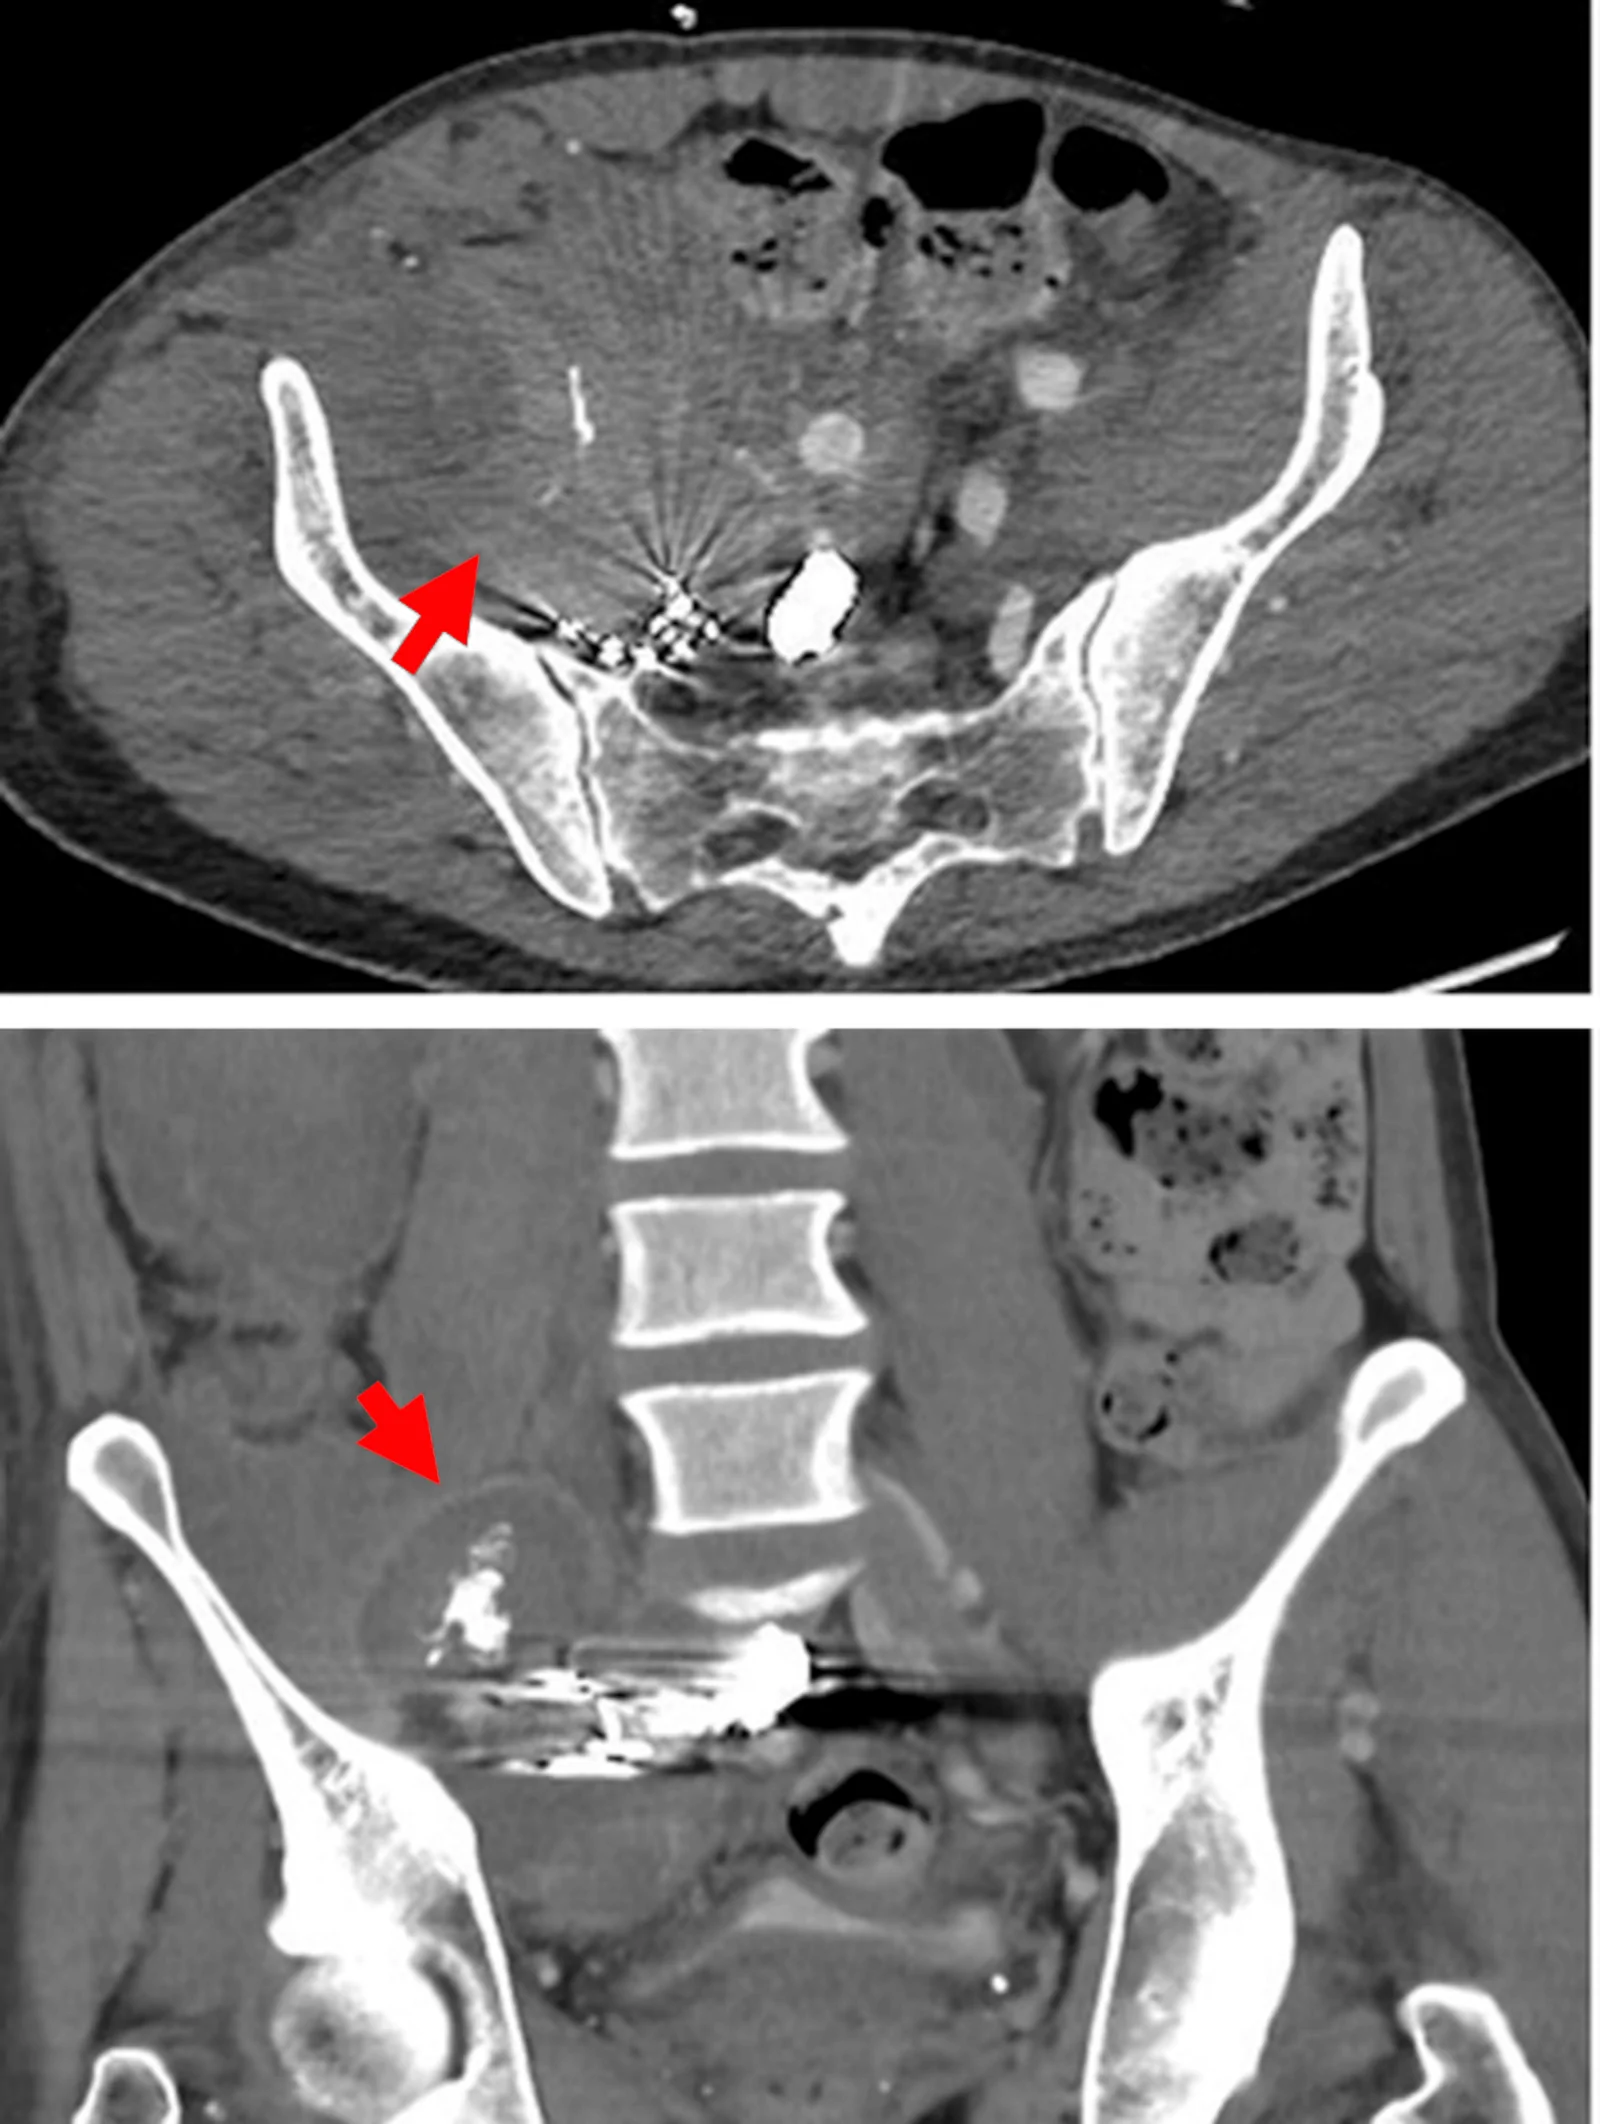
Postoperative computed tomography after endovascular repair CT: computed tomography; NBCA: n-butyl-2-cyanoacrylate Contrast-enhanced CT obtained after combined coil and NBCA embolization, showing no significant enlargement of the retroperitoneal hematoma and no evidence of active contrast extravasation. The red arrow indicates the ruptured internal iliac artery aneurysm occluded by coil embolization and NBCA
